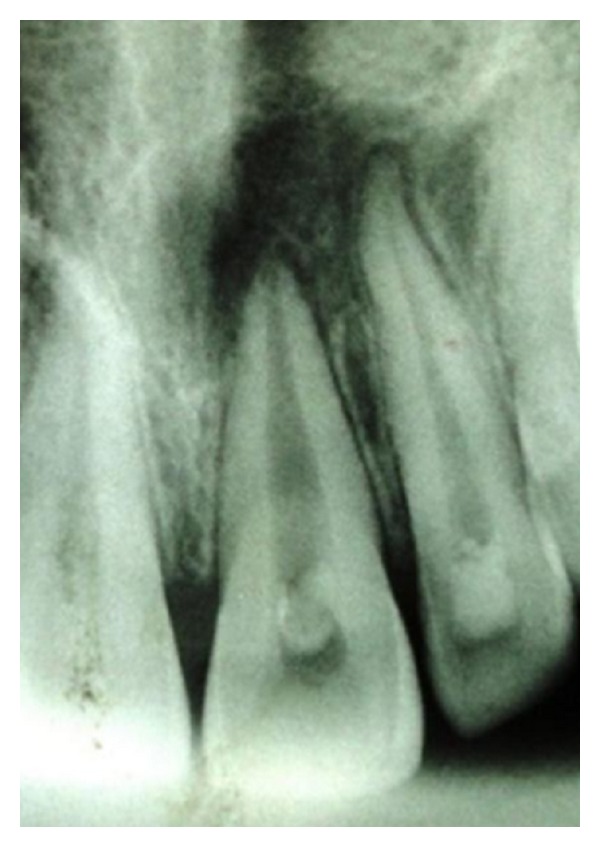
Preoperative radiograph of 21 and 22 showing a large periapical radiolucency.
